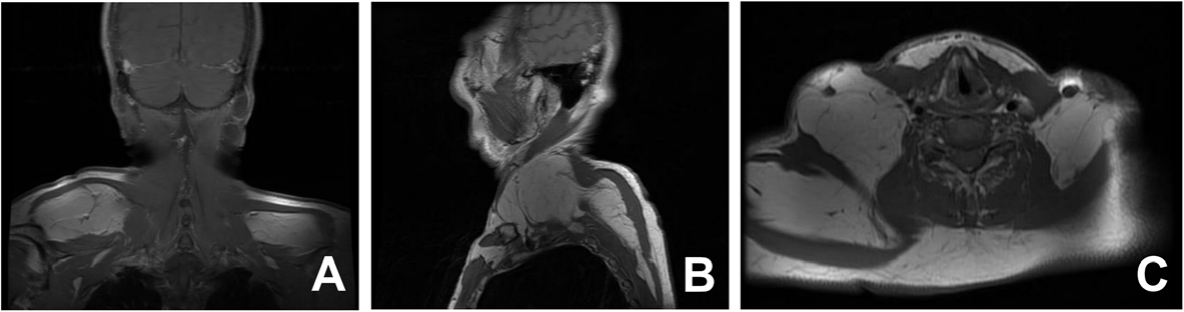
Magnetic resonance images of the patient’s neck. Coronal and sagittal T1 weighted sequences from the neck MRI revealed subcutaneous adipose tissue in both the shoulders (a) and posterior neck (b). Axial T1 weighted sequence findings also revealed diffuse subcutaneous fatty infiltration in both shoulders, but there was no mediastinal lipomatosis (c). MRI: Magnetic resonance image
